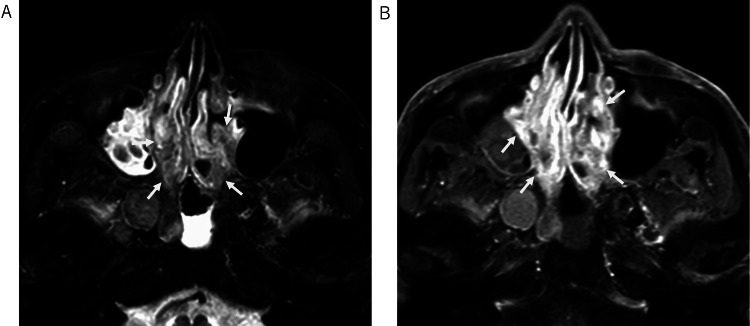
Nasal findings on MRI. Fat-saturated T2-weighted image reveals diffuse and irregular thickening of the nasal mucosa, containing interspersed areas of low signal intensity (A). Extensive enhancement is observed after contrast administration on post-contrast T1-weighted image (B).
